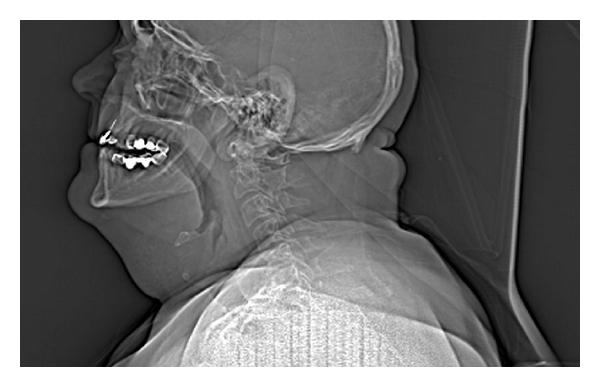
A scout CT view of the patient in case 2. This view shows a patent oropharyngeal airway despite superior and posterior displacement of the tongue secondary to a significant submandibular inflammation and swelling.
